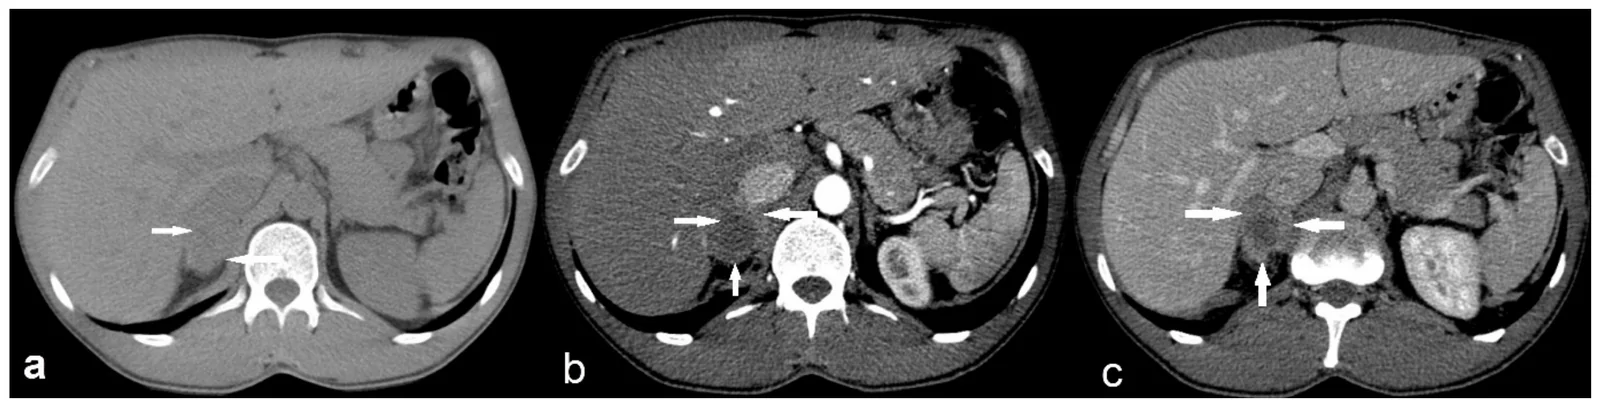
First follow-up CT axial images: (a) pre-contrast, (b) arterial phase, (c) venous phase showing the adrenal lesion decreased in size, with central hypodensity and peripheral contrast enhancement (arrows).
